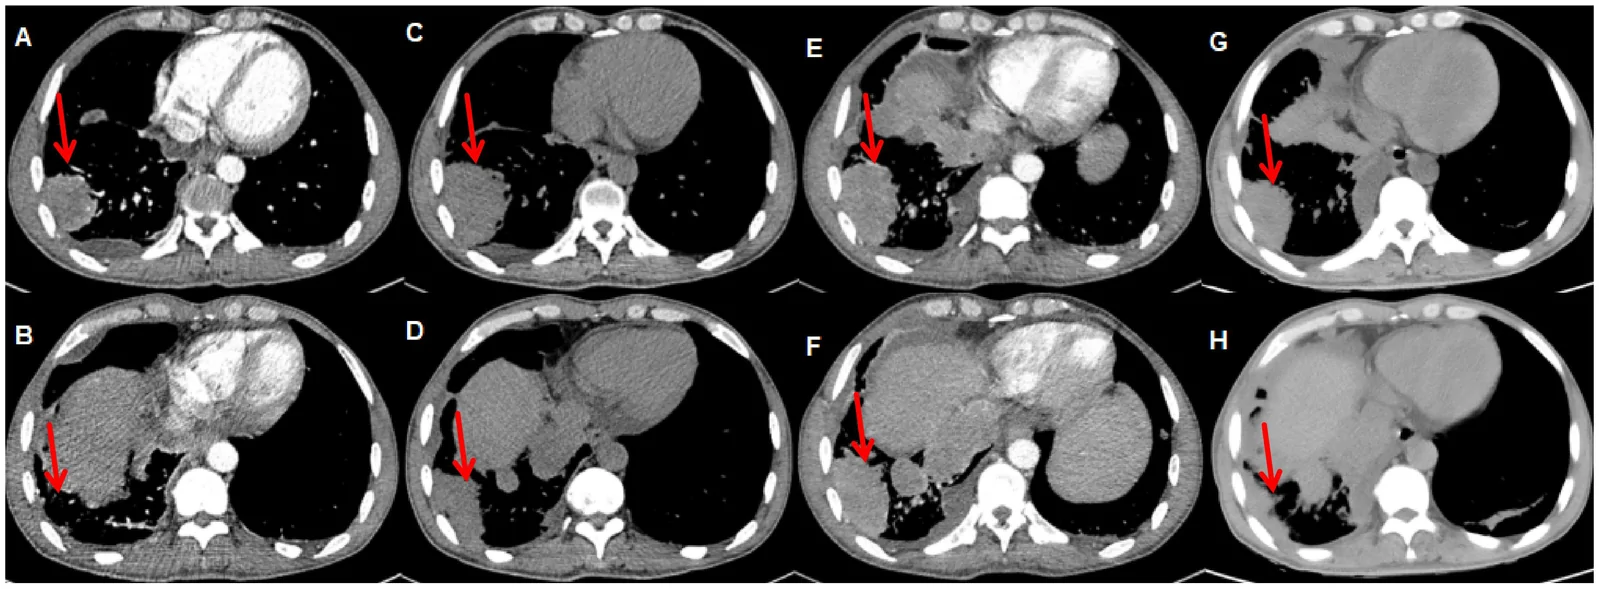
Serial chest CT images during the disease course and therapeutic response. Before treatment (A, B), the target lesion was a 43×33 mm right lower lobe mass, with non-target lesions including pleural nodules and lymphadenopathy. After one cycle of gemcitabine, nedaplatin, and camrelizumab (C, D), the target lesion increased to 62×40 mm (Progressive Disease). Following one cycle of liposomal paclitaxel and nedaplatin plus camrelizumab (E, F), further progression to 62×45 mm was observed (Progressive Disease). After two cycles of liposomal paclitaxel/cisplatin/endostar (G, H), the target lesion was 50×33 mm with a new left lower lobe lesion (Progressive Disease).
